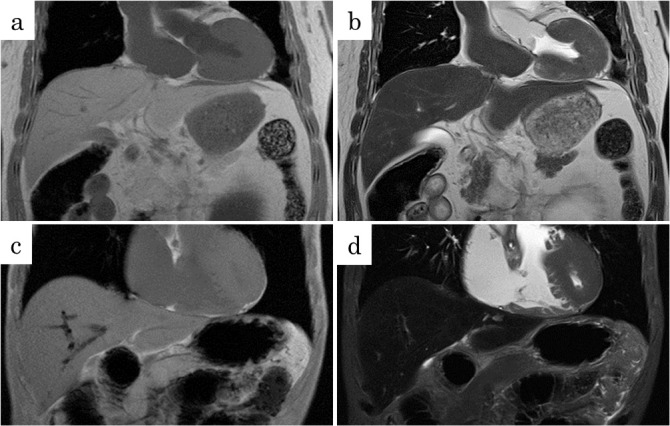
(a) Coronal T_1_-weighted image of the liver on postmortem magnetic resonance imaging (MRI). T_1_ value of the anterior segment of the right lobe and lateral segment of the left lobe is 474.3 and 571.9 ms (rectal temperature: 27.5°C). (b) Coronal T_2_-weighted image of the liver on postmortem MRI. T_1_ value of the anterior segment of the right lobe and lateral segment of the left lobe is 57.7 and 54.0 ms (rectal temperature: 27.5°C). (c) Coronal T_1_-weighted image of the liver on postmortem MRI. T_2_ value of the anterior segment of the right lobe and lateral segment of the left lobe is 500.5 and 401.5 ms (rectal temperature: 5.0°C). (d) Coronal T_2_-weighted image of the liver on postmortem MRI. T_2_ value of the anterior segment of the right lobe and lateral segment of the left lobe is 29.9 and 26.8 ms (rectal temperature: 5.0°C).
